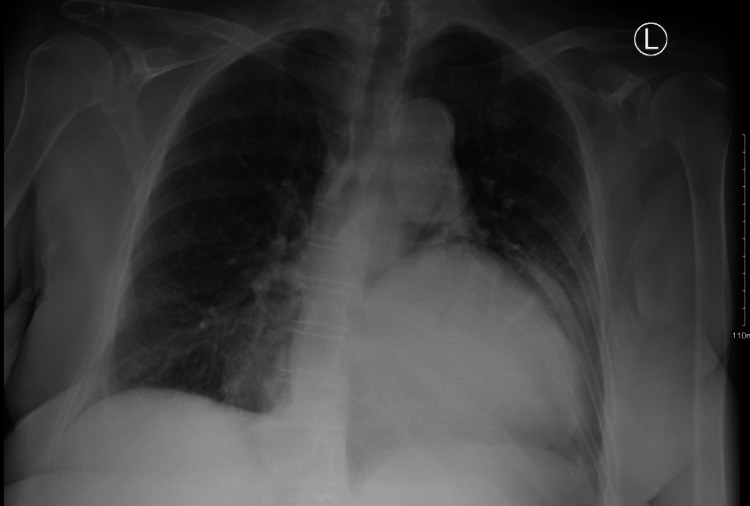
Radiograph chest posteroanterior (PA) view shows a left basilar opacity representing bowel gas suggestive of a hiatal hernia
